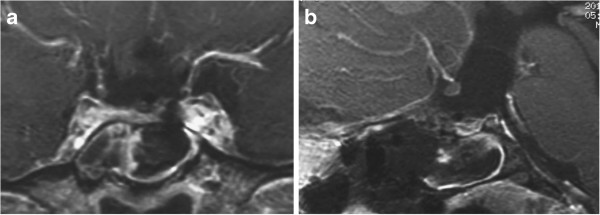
Case 2. Postoperative coronal (a) and sagittal (b) MR images revealing gross total removal of the tumor.
